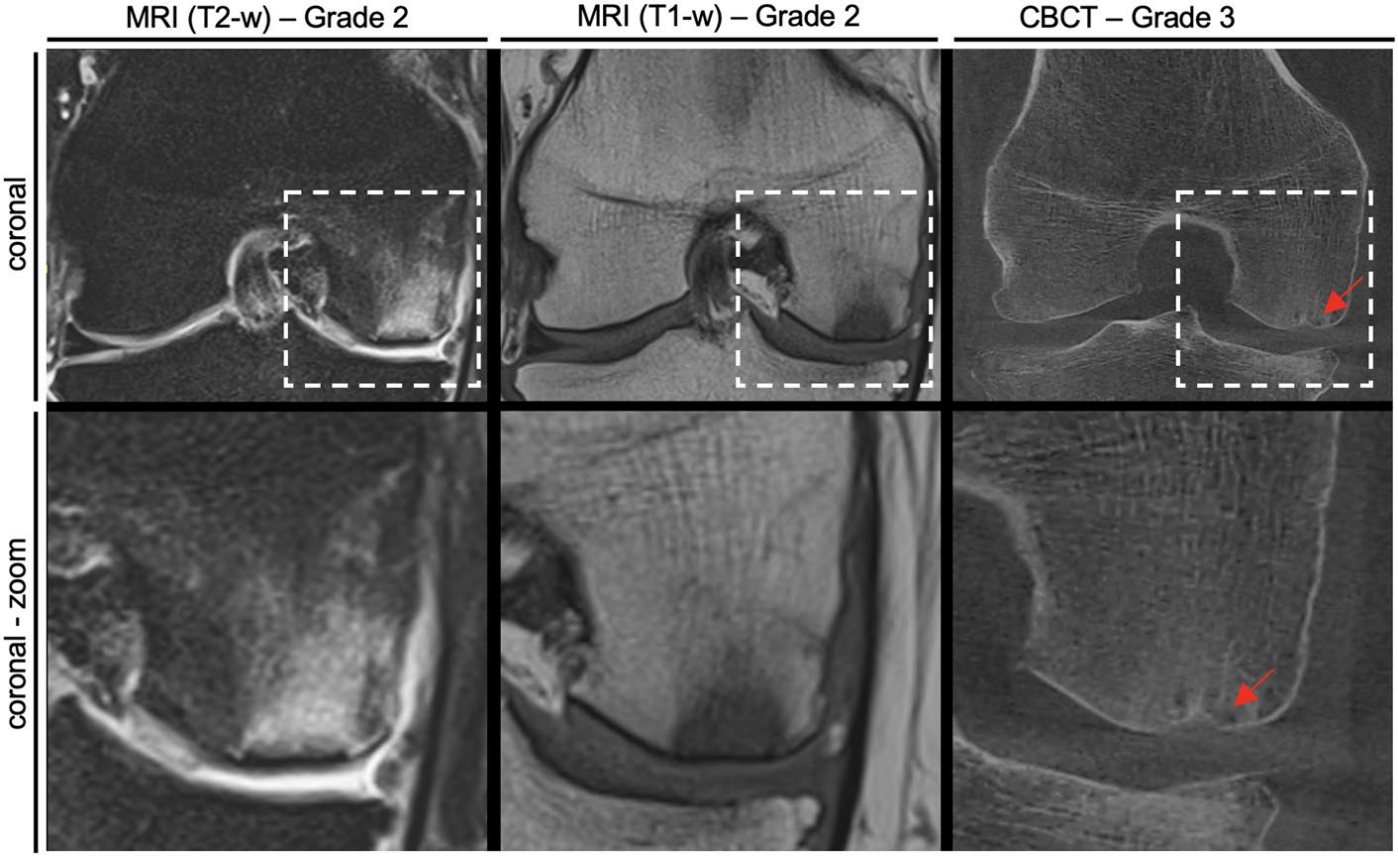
Representative case of a 61 year-old man with differing subchondral fracture gradings on MRI and CBCT. *MRI* magnetic resonance imaging, *CBCT* cone beam computed tomography. Note the grade 2 MRI findings of diffuse bone marrow edema, suspected subchondral fracture and cortical irregularities but without a circumscribed osseous infraction of the subchondral bone plate. Finally, CBCT performed three days after MRI unmasks a cortical step off with an infraction of the subchondral bone plate, suggesting a subchondral fracture grade 3 lesion.
